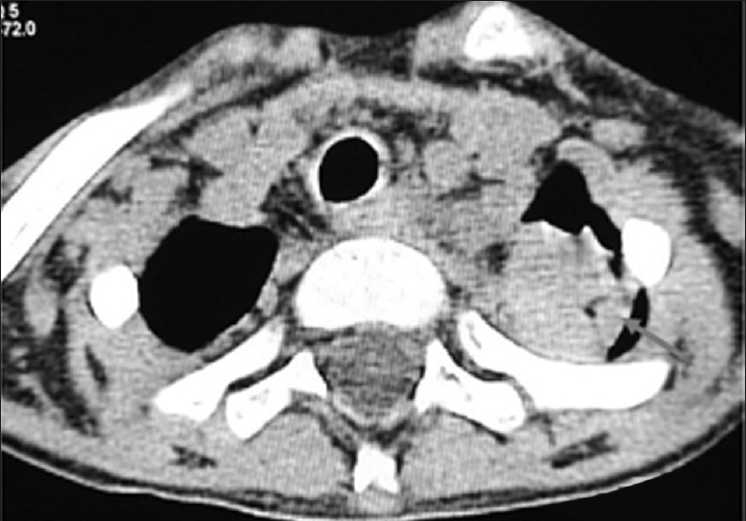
HRCT chest (mediastinal window) showing left apical mass with air crescent sign (arrow) and mediastinal invasion
